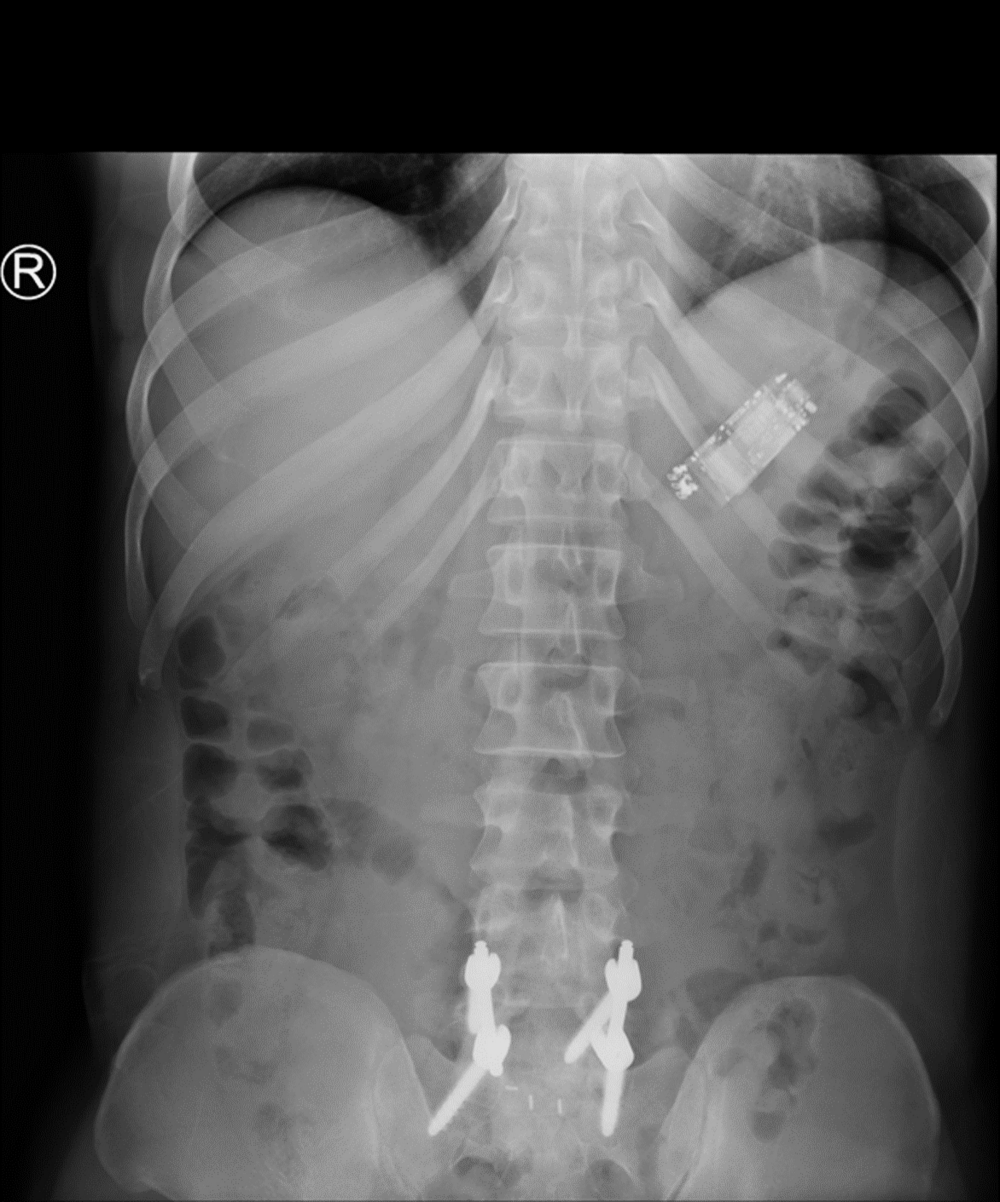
Initial KUB (abdominal plain X-ray). Revealed a radiopaque foreign body that appeared to be in the stomach. The patient was made NPO and general surgery was consulted. KUB, kidneys, ureters, and urinary bladder; NPO, nil per os.
